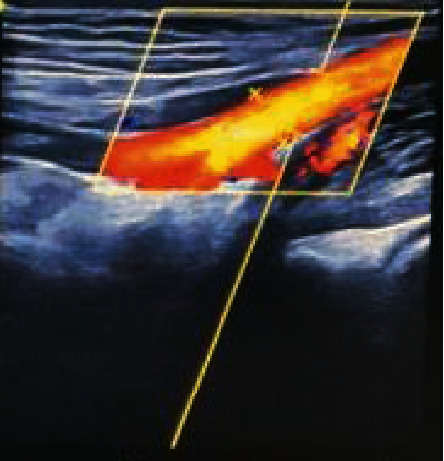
Brachial artery colour Doppler images.
